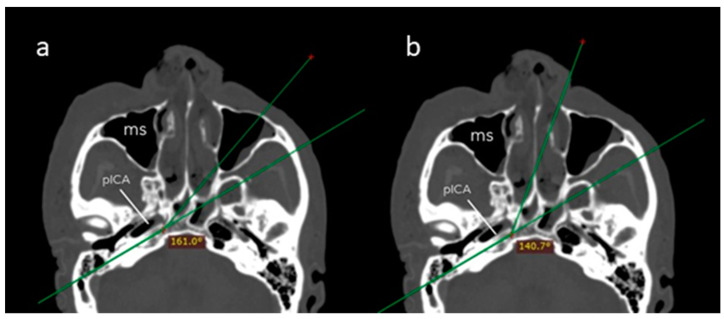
Axial CT scan showing the angle of attack of the endoscopic endonasal (a) and contralateral transmaxillary (b) approaches. A line parallel to the axis of the petrous segment of the internal carotid artery is used as a reference to calculate the angles of attack. MS: maxillary sinus.
